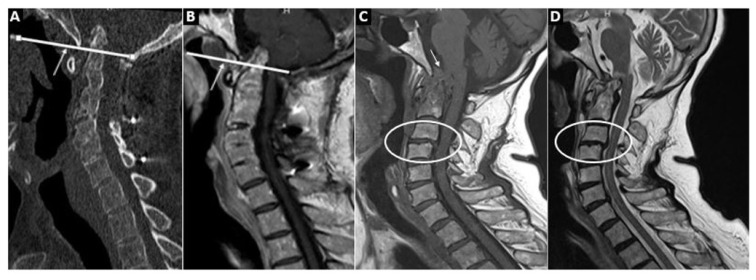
A (cervical CT) and B (cervical MRI) show the Chamberlain line and the associated vertical subluxation. In C (T1WI) and D (T2WI), basilar impression with pannus tissue at the craniovertebral junction causing stenosis of the foramen magnum and compression of the brainstem are shown. In addition, subaxial subluxation between C3–4 is seen.
